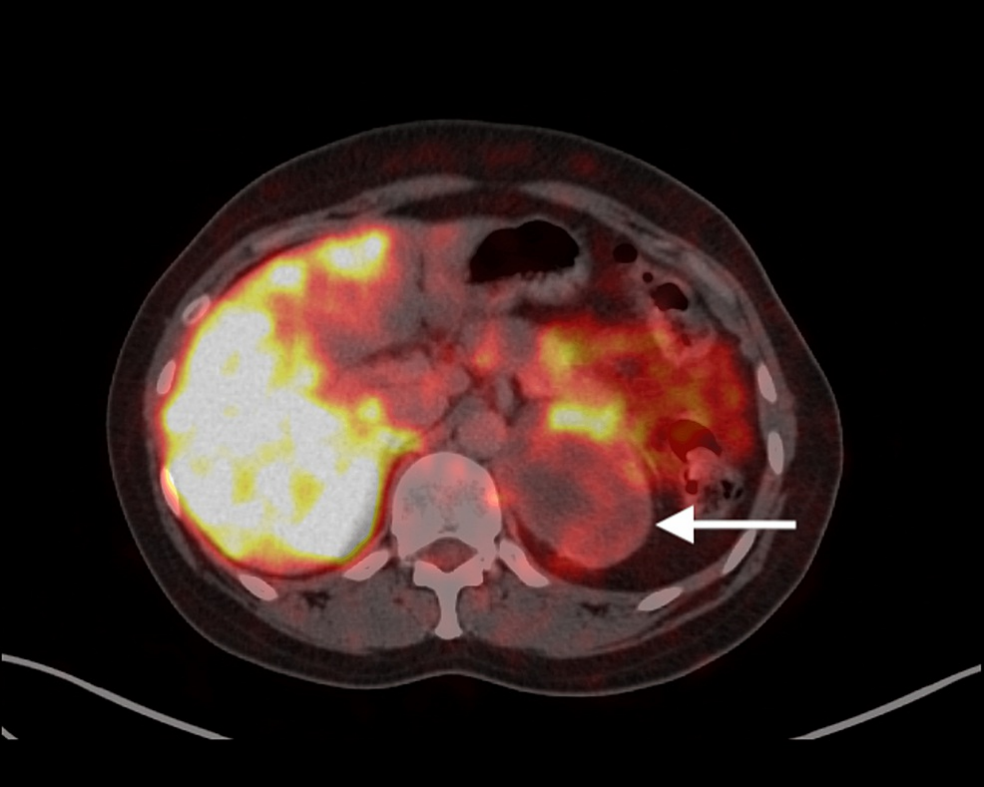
Axial section of fluorodeoxyglucose positron emission tomography (FDG PETCT) showing faint tracer uptake of the left suprarenal mass (white arrow).
